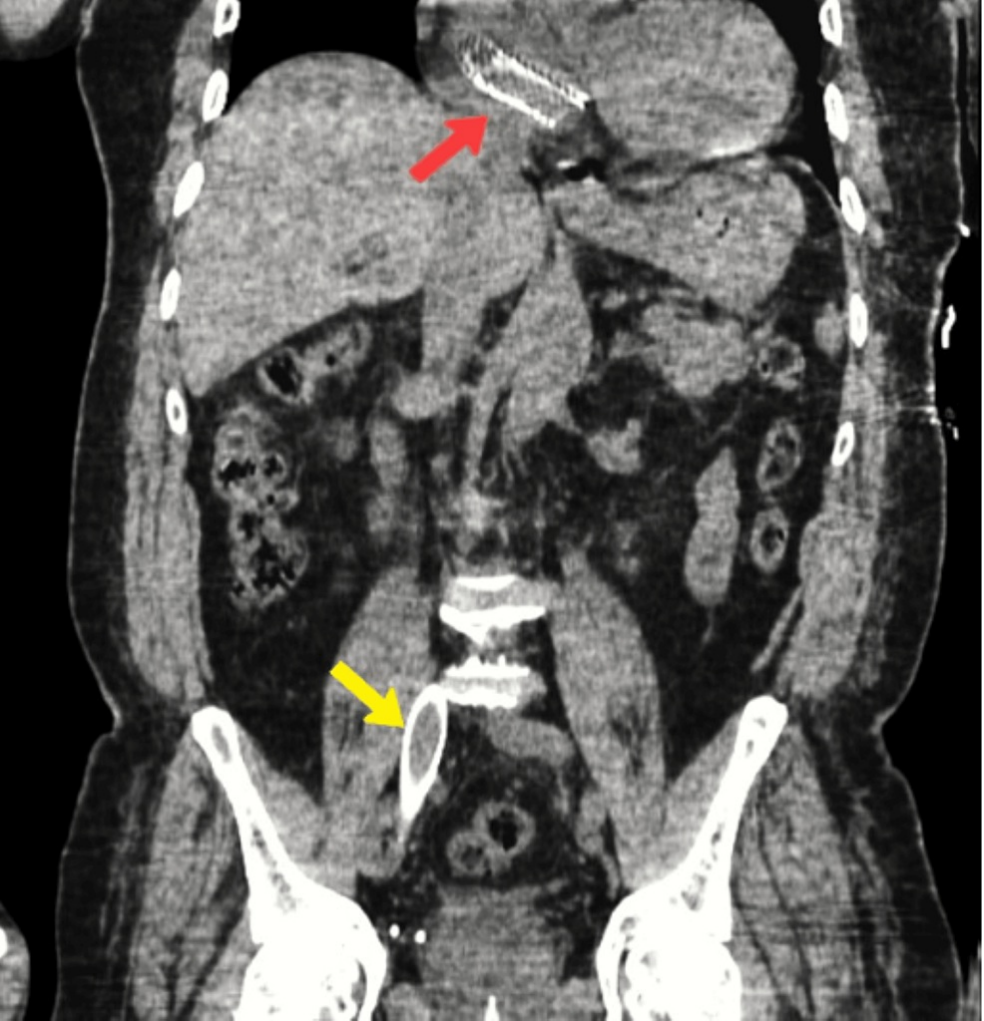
CT of the abdomen (coronal plane). Red arrow pointing at the migrated stent in the right atrium. Yellow arrow pointing at the right common iliac vein stent in place
